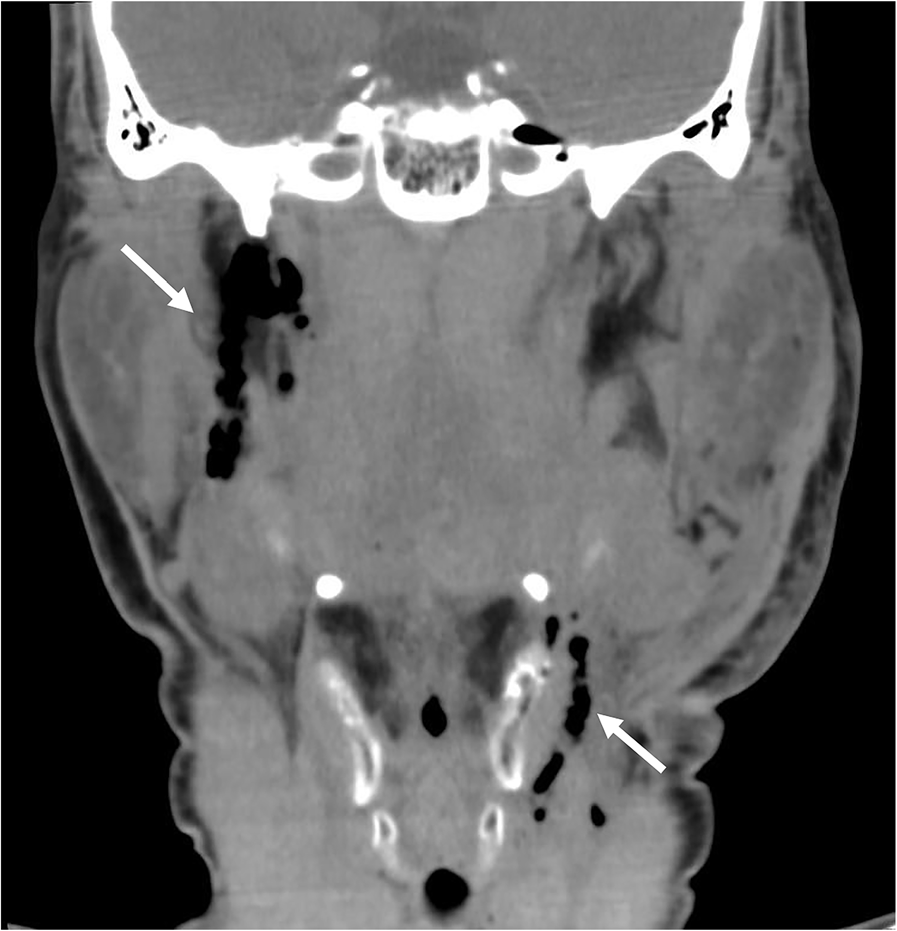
Computed tomography scan showing air in the regions of the left mandible and right styloid process of the temporal bone (*white arrows*), suggesting a penetrating neck injury extending to the skull base
